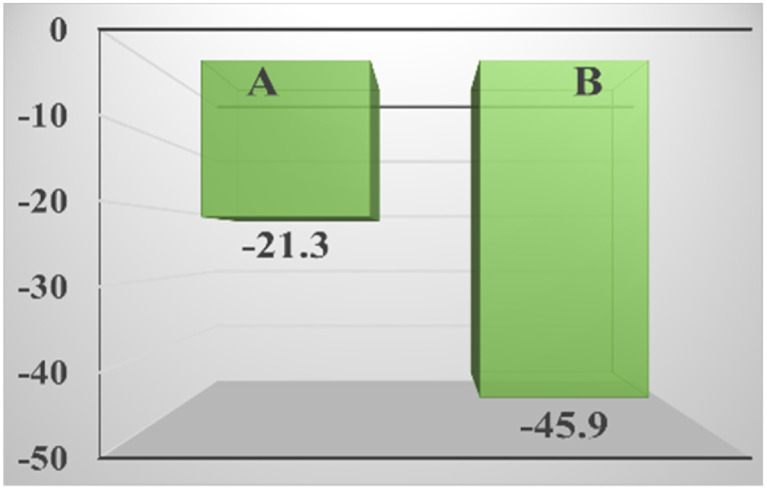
Zeta potential of the zeolite loaded with silver by the impregnation method (A) and the zeolite loaded with silver in the strong ultrasound and electric field (B).
